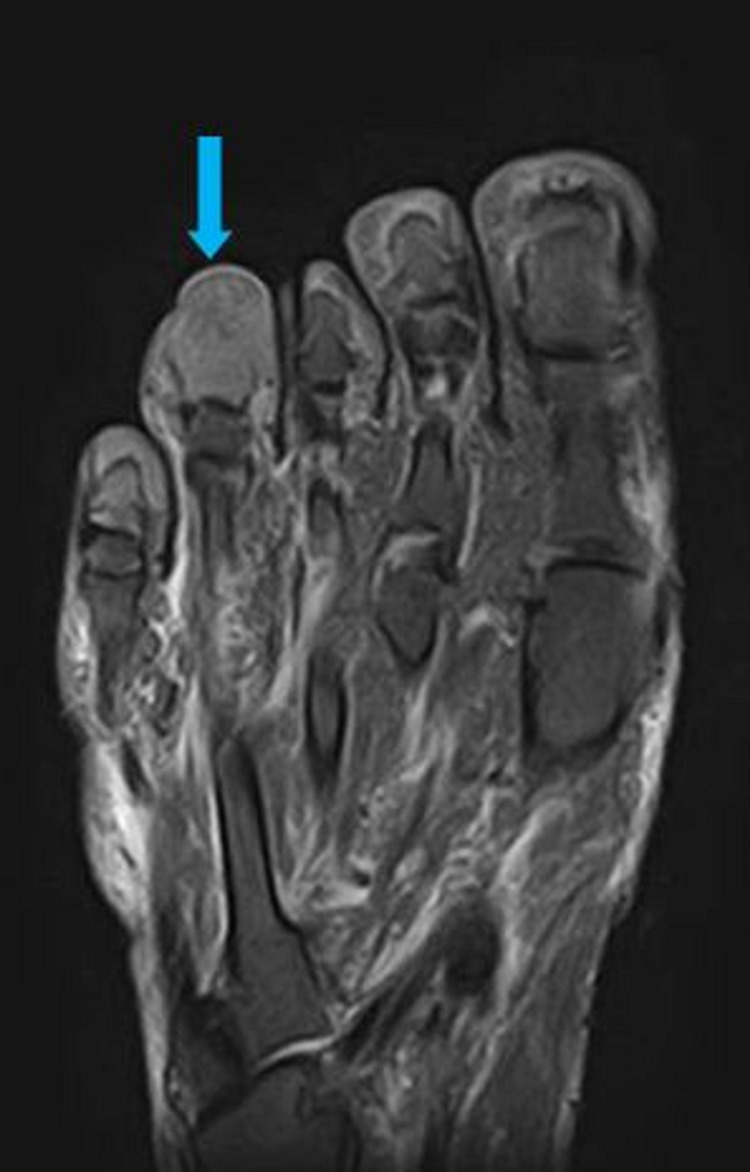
Coronal view on the MRI, T2 STIR sequence visualizing the mass on the distal phalange of the fourth toe (blue arrow) STIR, short tau inversion recovery
